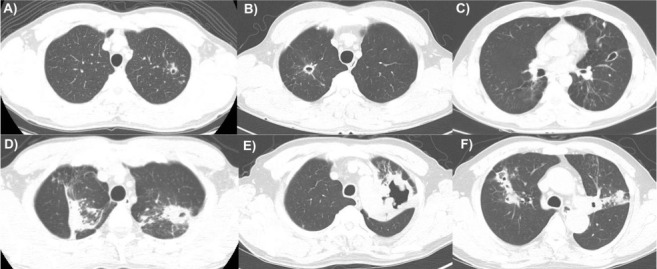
Characteristic CT findings of MDR-TB and DS-TB. A–C) DS-TB in a 52-year-old man. Chest CT axial images with lung window settings showed several cavities in both lungs. The average wall thickness of the cavities is approximately 6 mm. D–F) MDR-TB in a 62-year-old man. Chest CT axial images with a lung window setting showed six or more cavities in both lungs. The walls were relatively thick (>11 mm). CT = computed tomography; MDR-TB = multidrug-resistant TB; DS-TB = drug-susceptible TB.
